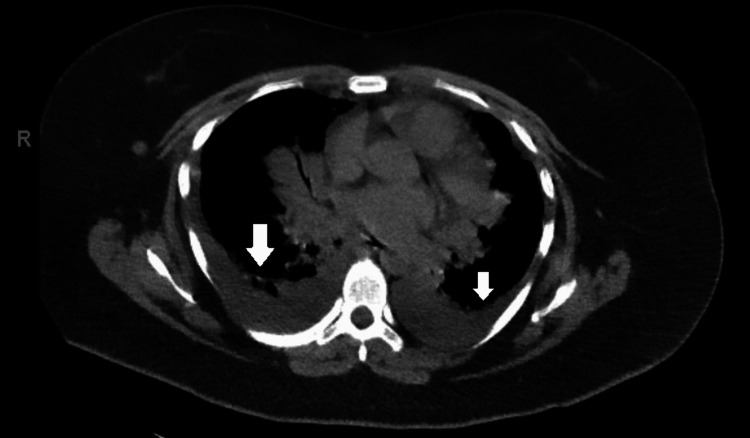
Computed tomography of the chest without contrast with arrows depicting small bilateral pleural effusions.
